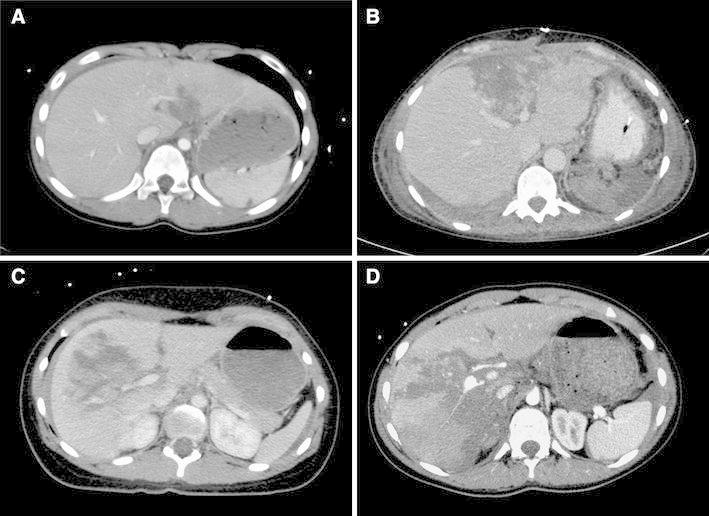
Representative CT scans in axial projections of two patients with type A (a, b) and two patients with type B (c, d) injuries following blunt liver trauma. The patient with the type B liver rupture was managed conservatively despite massive destruction of the hepatic parenchyma corresponding to a grade IV injury according to Moore score. The underlying traumas in these patients were a fall from a 3 m height onto the abdomen, b a rear-end collision, c a side-impact car crash, d a fall from a 10 m high climbing scaffold
